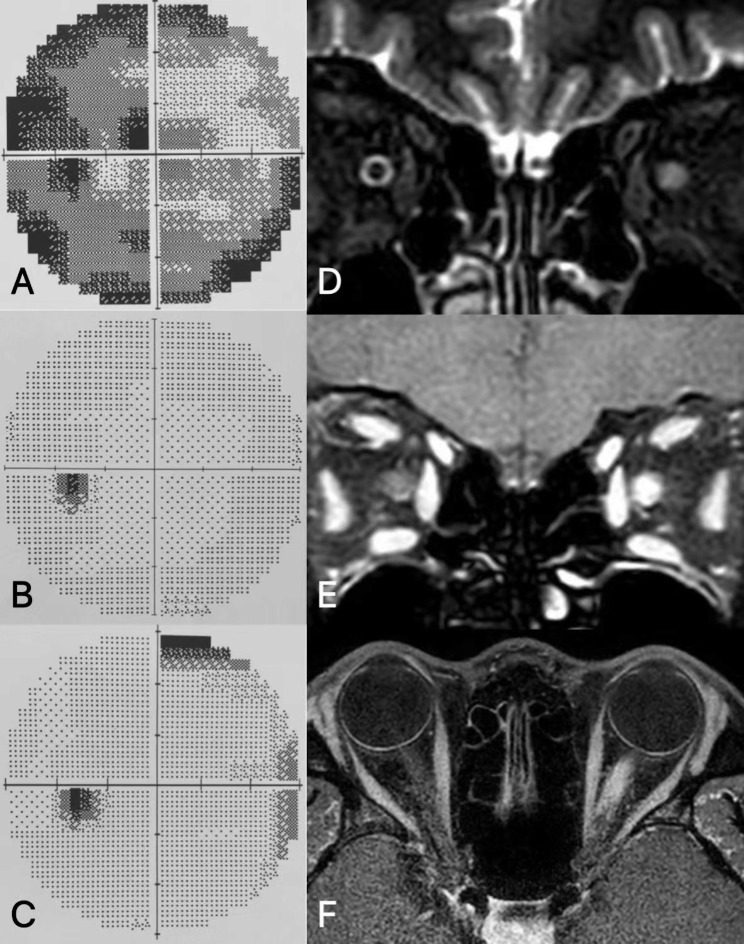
Evolution of visual field and optic nerve MRI in Patient 1. Automated visual field tests showed diffuse defects on day 7 after onset (A) that then resolved to normal spontaneously 2 weeks later (B). Seven weeks later, there were peripheral visual field defects when disease relapse occurred (C). MRI was performed on day 13 following onset, and the patient was not treated with corticosteroids before the scan. MRI revealed left optic nerve enlargement and hyperintensity in the orbital segment on coronal short tau inversion recovery image (D) and enhancement on postcontrast coronal (E) and axial T1 (F).
